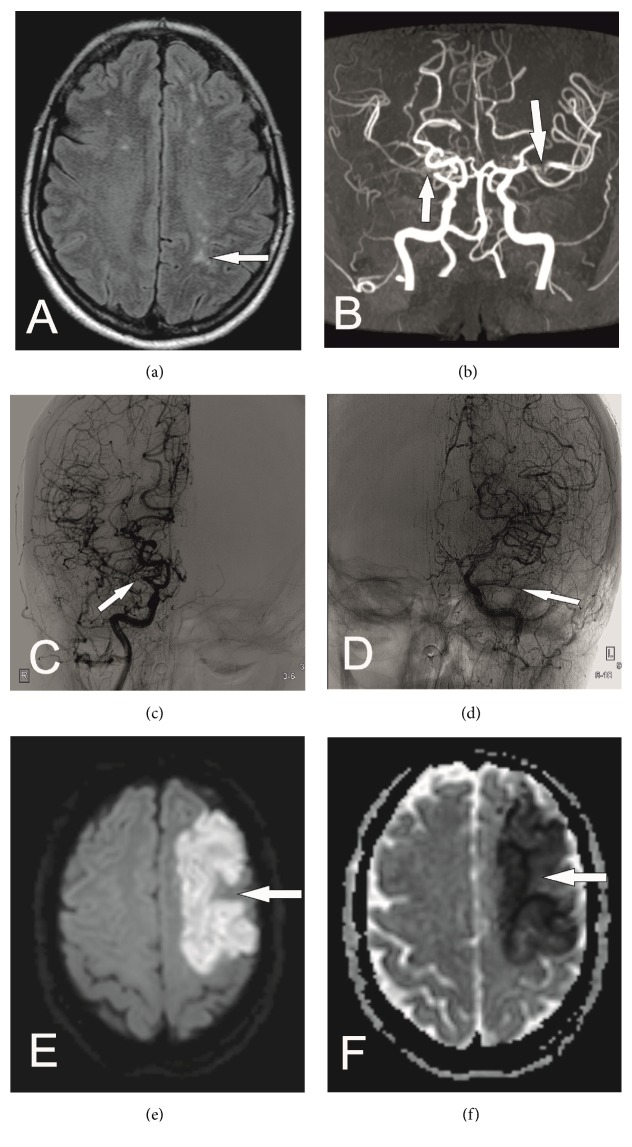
(a) Axial FLAIR image reveals multiple hyperintensities in watershed territories. (b) Time of Flight MRA shows bilateral MCA occlusions (arrows). (c, d) DSA of the right (c) and left (d) internal carotid arteries verifies the MCA occlusions (white arrows). Proliferation of small abnormal net-like vessels is also noticed. Diffusion Weighted Imaging (e) and Apparent Diffusion Coefficient (ADC) map (f) reveal diffusion restriction in the left MCA territory due to an acute infarct (arrows).
